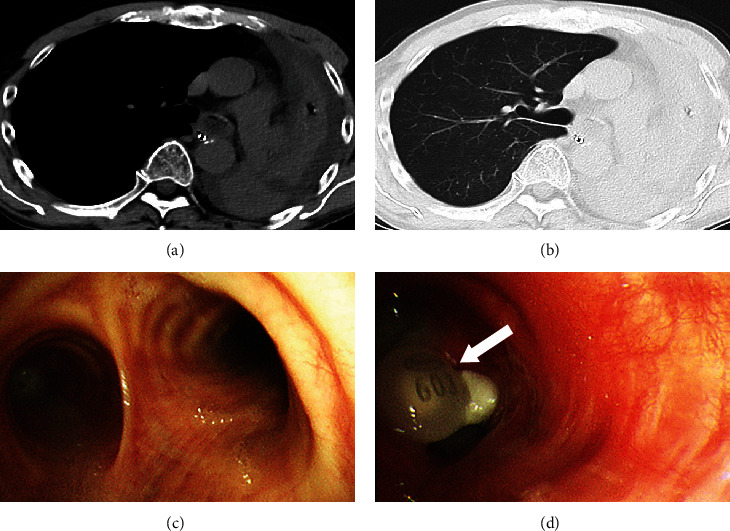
(a, b) Chest computed tomography images demonstrating sputum accumulation in the left main bronchus and a completely atelectatic left lung. Bronchoscopic images showing sputum obstruction of the (c) left main bronchus and the aspirated valproic acid tablet (arrow) in the (d) left inferior lobar bronchus.
